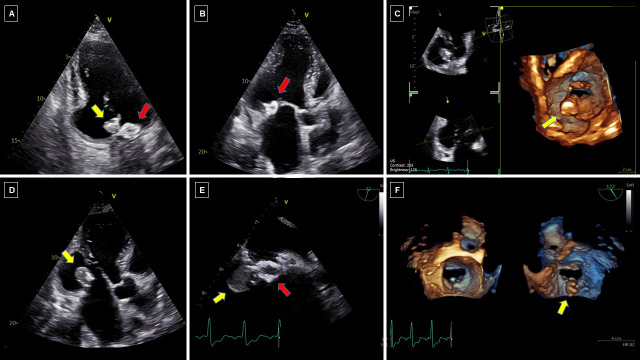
Echocardiography of ruptured CCMA with leaking caseous material. Two-dimensional (A,B,D,E) and three-dimensional (C,F) transthoracic (A–D) and transesophageal (E,F) images in a 69-year-old man with previous inferolateral ST-segment elevation myocardial infarction treated by percutaneous coronary intervention with stent implantation on left circumflex artery, showing an intracardiac mass suspected for leaking caseous material.
